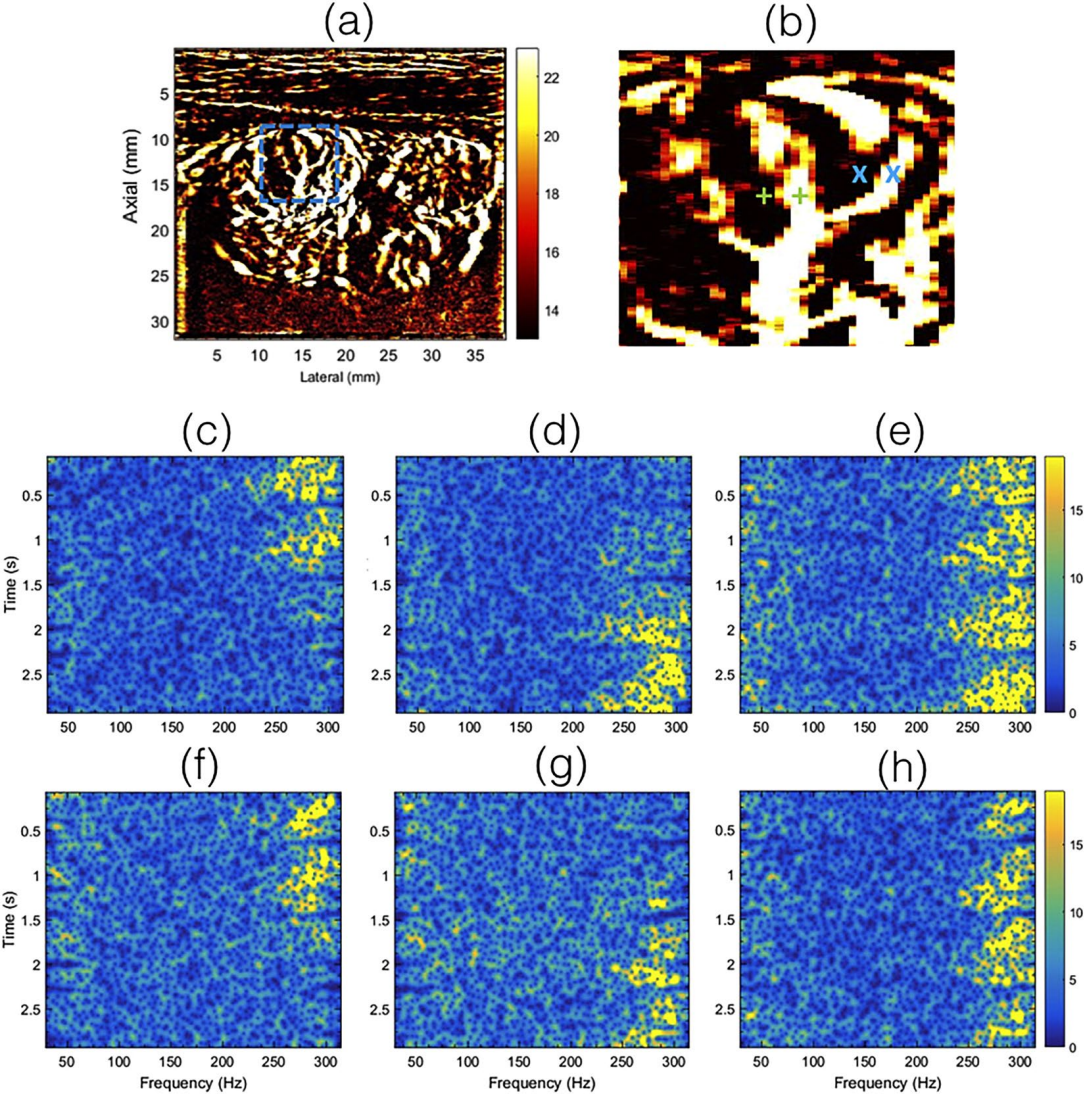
Displays the time-frequency spectrogram associated with the clutter filtered Doppler ensemble, prior to PD computation. Each time-frequency spectrum was calculate at a single-pixel spatial location, indicated in zoomed inset (b), obtained from the PD image (a). Specifically, figures (c–e) and (f–h) corresponds to the green ‘+’ and blue ‘x’ in (b), respectively. The time-frequency spectrums displayed in (c,f) and (d,g) were obtained without motion correction. Data displayed in (c,d) corresponded to the locations indicated by the left and right green ‘+’ in (b), respectively, prior to motion correction. Similarly, the data displayed in (f,g) corresponded to the locations indicated by the left and right blue ‘x’ in (b), respectively, prior to motion correction. Each of the green ‘+’ and blue ‘x’ pixel pairs were separated by 4 pixels. The time-frequency spectrums for the motion corrected data were displayed in (e,h), obtained from the location indicated by the right ‘+’, ‘x’ markers in (b), respectively.
